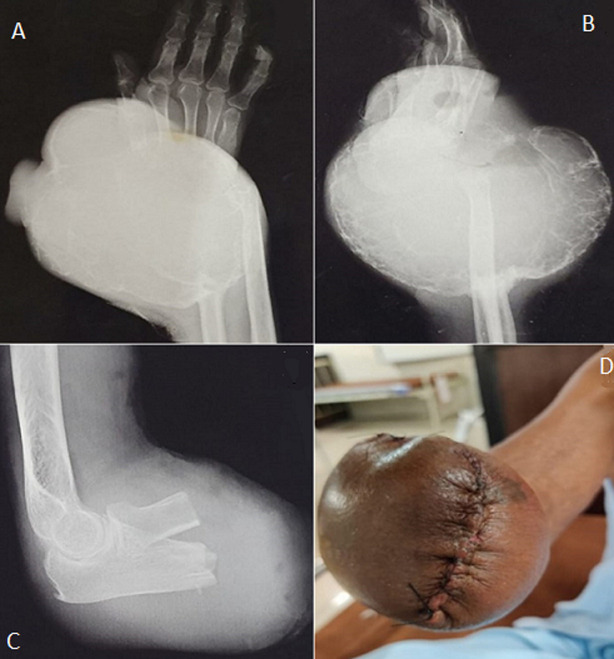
X-ray of right wrist showing giant cell tumor: A) anteroposterior view, B) lateral view; C) post-operative X-ray of elbow amputation; D) amputated limb with sutures
